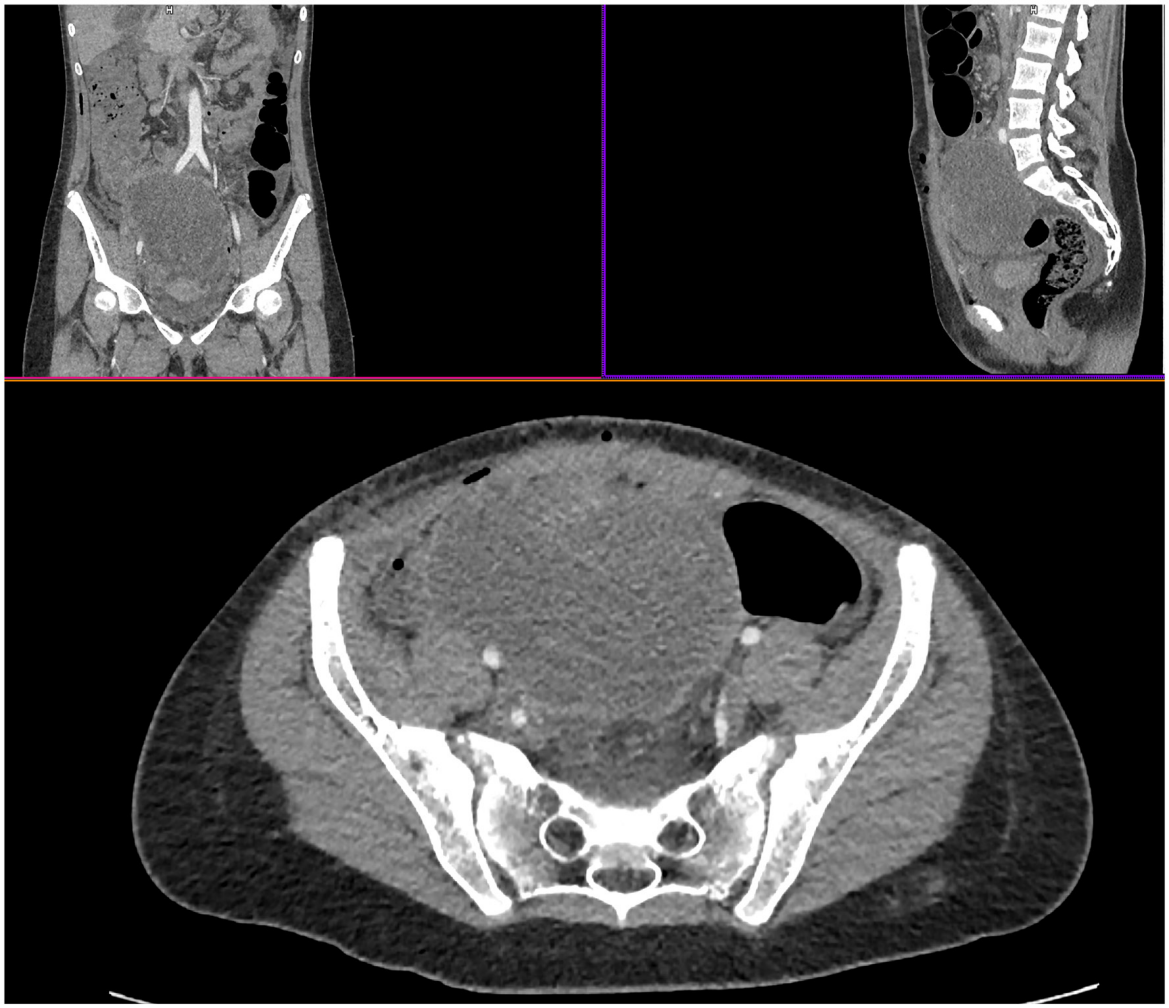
A multislice computed tomography of the original tumor. The image shows a distinctly outlined, moderately contrast-accumulating entity in the pelvic cavity at L5-S4 spinal level, without clear organ affiliation, causing displacement of adjacent structures.
